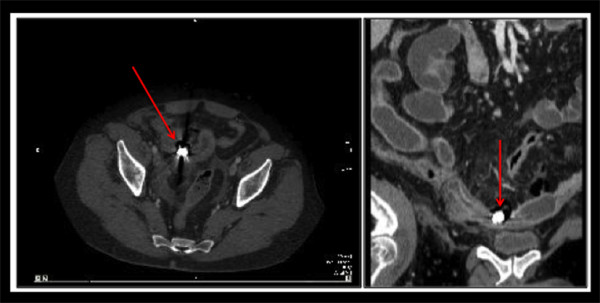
Computed tomography scan of the abdomen in case 2. Scan shows the presence of the capsule endoscope located in the distal ileum (red arrows), stricture of the small bowel along with extensive small bowel distension, a thickened bowel wall and loculated free air.
